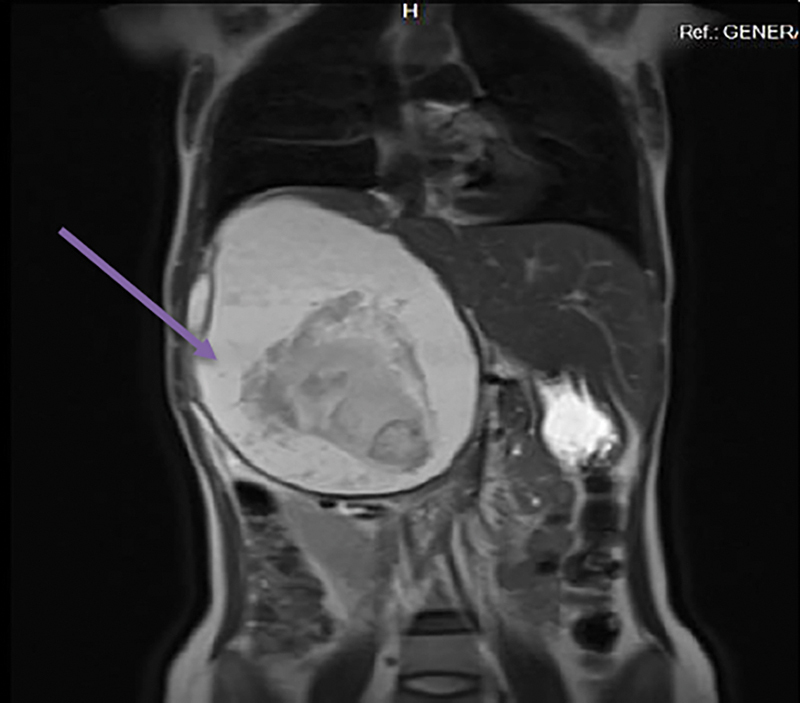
Magnetic resonance imaging coronal section showing a large right hypochondrial mass pushing the diaphragm and indenting on the liver surface along with a variable tissue appearance inside.
